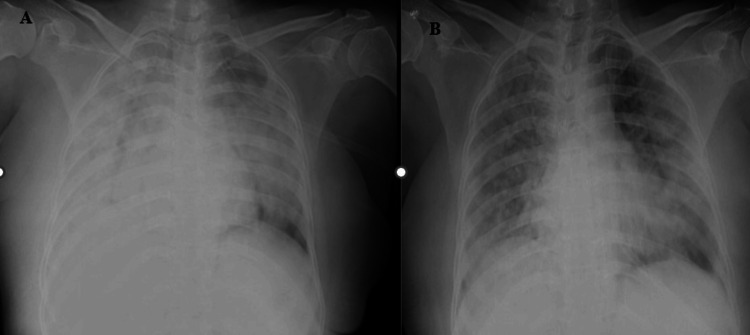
Figure 3A: The supine chest radiographs (anteroposterior (AP) view) of a 53-year-old male patient revealed diffuse ill-defined heterogenous opacities with few linear lucencies likely air bronchogram suggestive of consolidations in bilateral lung parenchyma involving > 75%. Radiographic disease severity score was eight (severe grade). Figure 3B: Follow-up AP supine chest radiographs of the same patient after seven days revealed areas of consolidations and ill-defined nodules in bilateral lung parenchyma involving > 75%.
